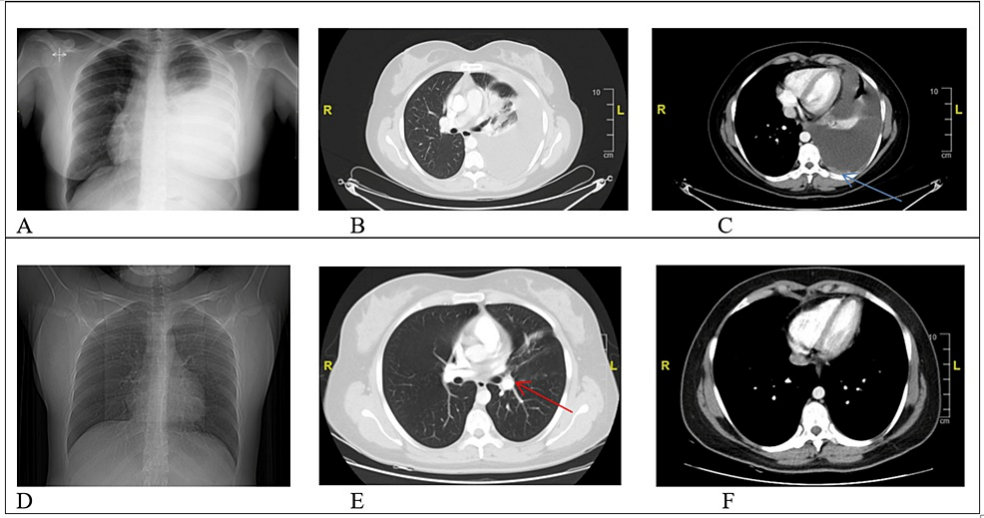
Chest X-ray and CT sections before crizotinib treatment (A, B, C) and after crizotinib treatment (D, E, F) (A) left-sided massive pleural effusion and right-sided mediastinal shift on chest X-ray; (B) and (C): left-sided massive pleural effusion and pleural metastasis (blue arrow) are remarkable on CT sections; (D): left-sided massive pleural effusion completely disappeared on chest X-ray; (E) and (F): massive pleural effusion and pleural metastasis disappeared on CT sections. Also, a primary tumor with a diameter of 2 cm in the left lung (red arrow) became detectable.
